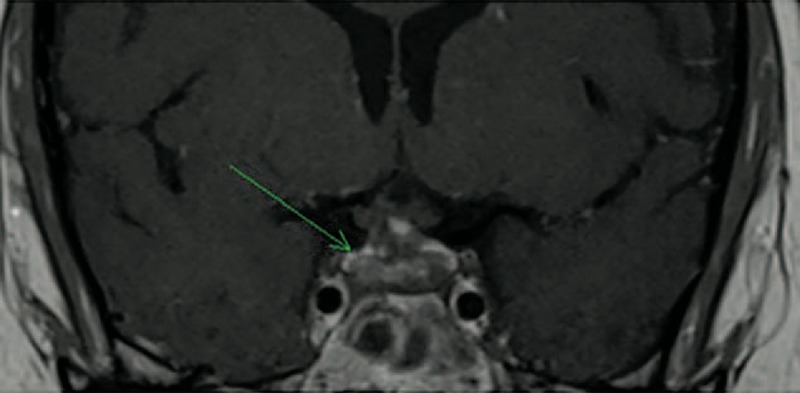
Patient 3 in 2011: coronal brain T1-weighted MR image after intravenous administration of gadolinium showing reduction in size of pituitary with heterogeneous enhancement.
